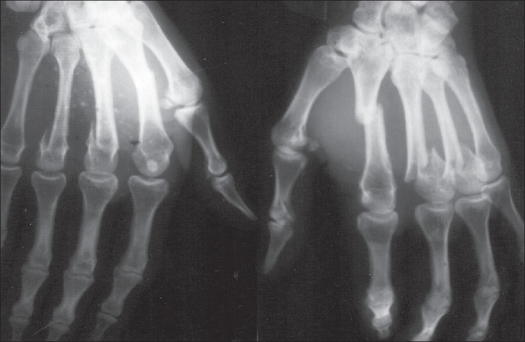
Preoperative radiographs showing transverse fracture of 2nd metacarpal; and fracture neck of 3^rd^ and 4^th^ metacarpals
